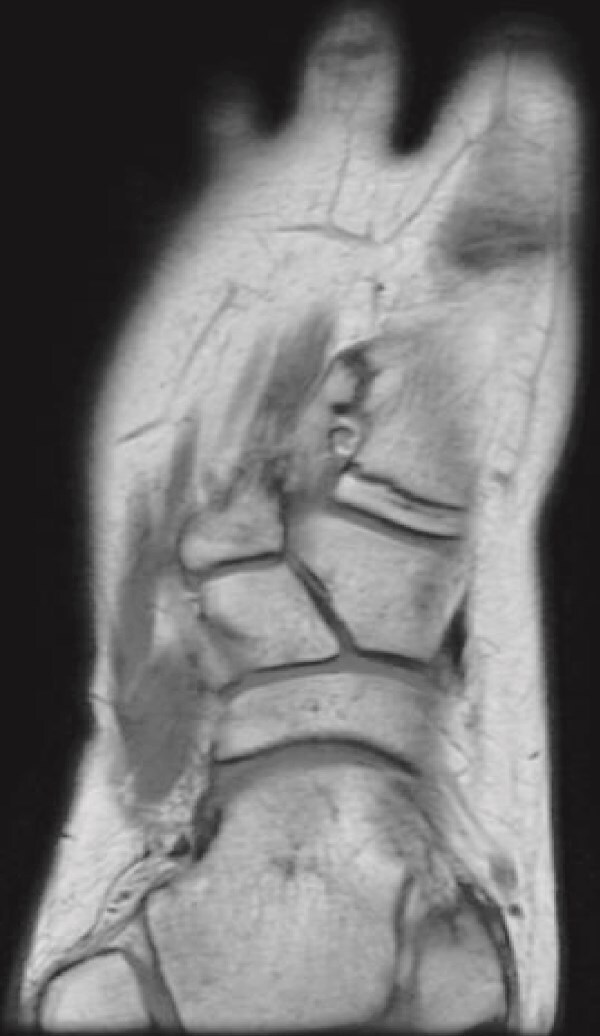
Coronal T1-weighted MRI of the right foot showing a rudimentary bone interposed between the first and second metatarsals with pseudo-articulation and ankylosis with the midshaft of the first metatarsal.
